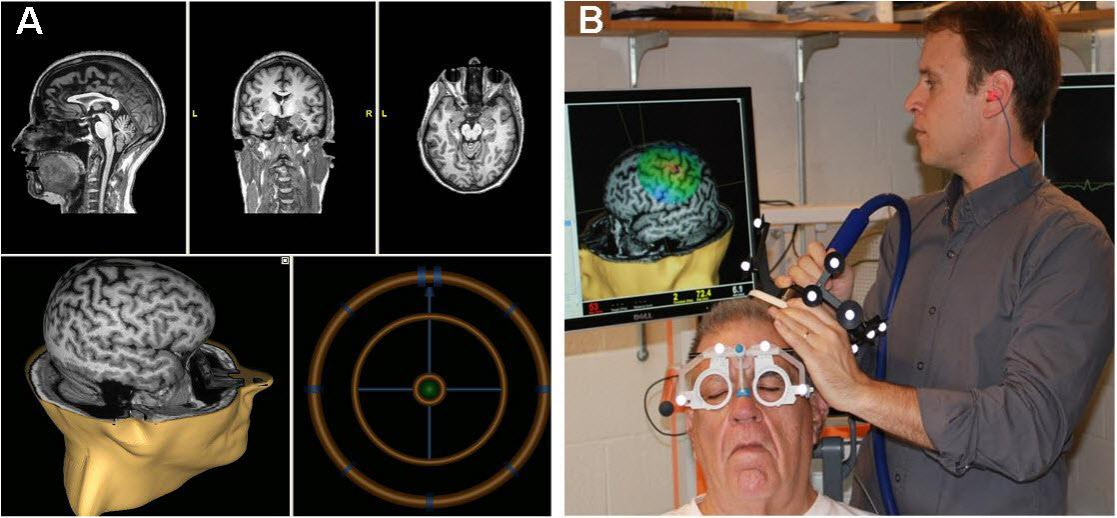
The MRI-navigated Nexstim Interface. A: Screen shot of the Nexstim neuronavigation interface: The top three panels represent the sagittal, coronal, and axial (left to right) MRI views used to locate specific spatial landmarks relative to the stereotactic spheres (shown in panel B) that are used for neuronavigated TMS. The lower panels display the stimulation target on a 3D MRI (left) and the bulls-eye target (right) that ensures that the operator holds the coil to the patient’s scalp at the correct location and orientation. B: The operator holds the figure-of-eight coil to the patient’s scalp while monitoring the brain stimulation site on the 3D MRI. Note the stereotactic spheres mounted on the coil that identify its position relative to the spheres on the patient’s goggles that localize the patient’s head.
